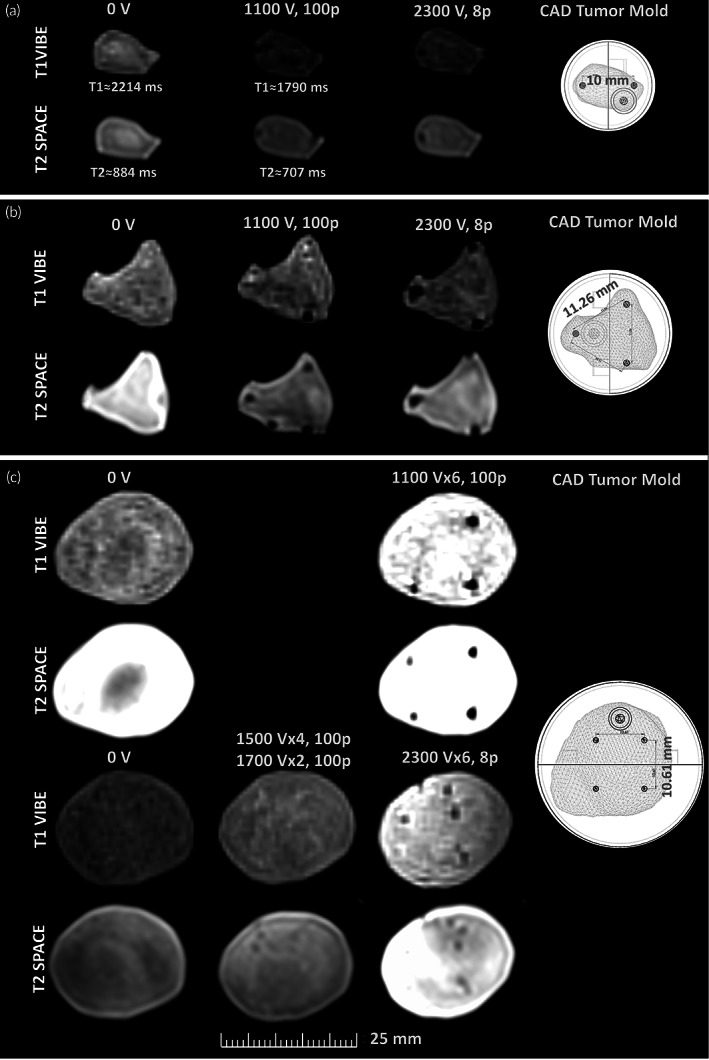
T1‐weighted VIBE and T2‐weighted SPACE MR images of (a) small, (b) medium, and (c) large tumor‐shaped hydrogels, 24 h after IRE. Images acquired along parallel and perpendicular orientation to the electrode insertion. The distance between the needle–electrodes appears in the CAD molds. Untreated tumors were utilized as control conditions. Small and medium treated tumors were hypointense in T1‐weighted VIBE images and hyperintense in T2‐weighted SPACE images, 24 h after IRE. Oppositely, inverting evolution of the SI of large tumors from hyperintense, within the first 24 h after IRE is visualized.
